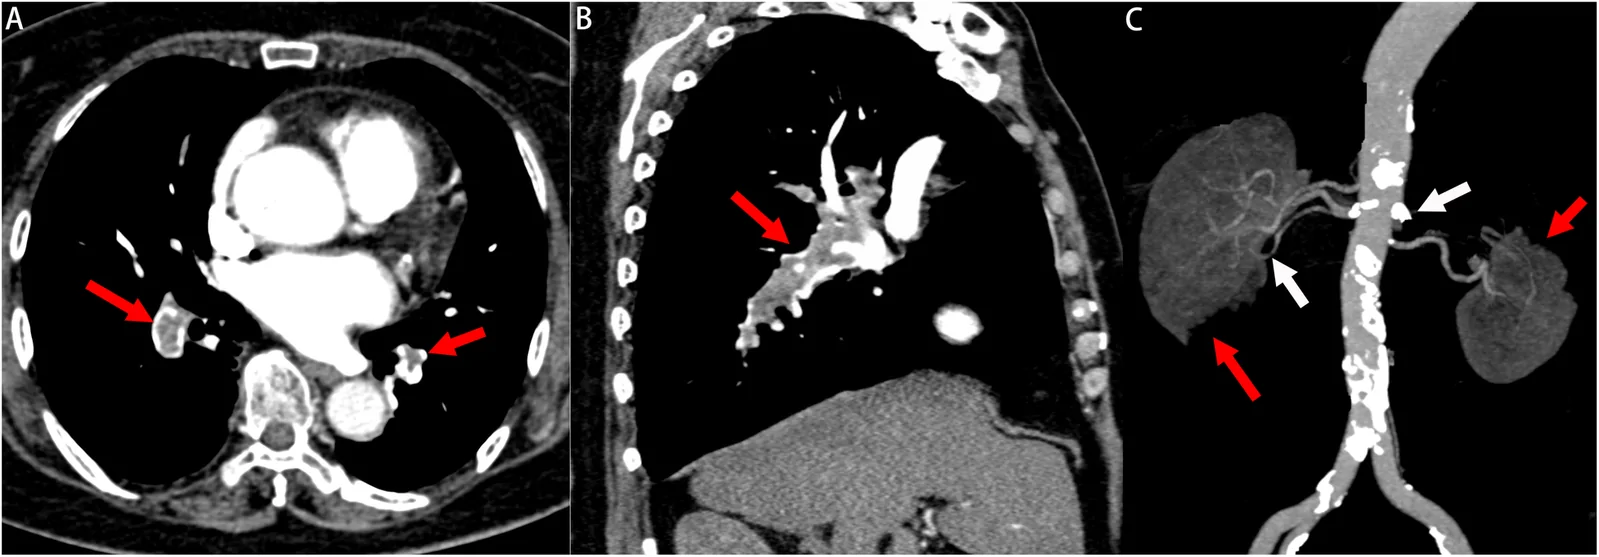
(A) axial pulmonary CTA demonstrated multiple intraluminal filling defects in the bilateral lower lobe pulmonary arteries (red arrows), consistent with acute pulmonary emboli. Markedly decreased peripheral pulmonary vascular opacification and sparse distal vascular branches were observed, indicating impaired pulmonary perfusion. (B) Multiplanar oblique sagittal reconstruction of pulmonary artery CTA showed extensive thrombotic filling defects involving the right main pulmonary artery and right lower lobar pulmonary artery (red arrows), with extension into segmental branches. (C) Mesenteric CTA revealed bilateral accessory renal arteries, with embolism of the left main renal artery and the right accessory renal artery (white arrows). Corresponding wedge-shaped perfusion defects were identified in the mid-to-upper pole of the left kidney and the lower pole of the right kidney (red arrows).
